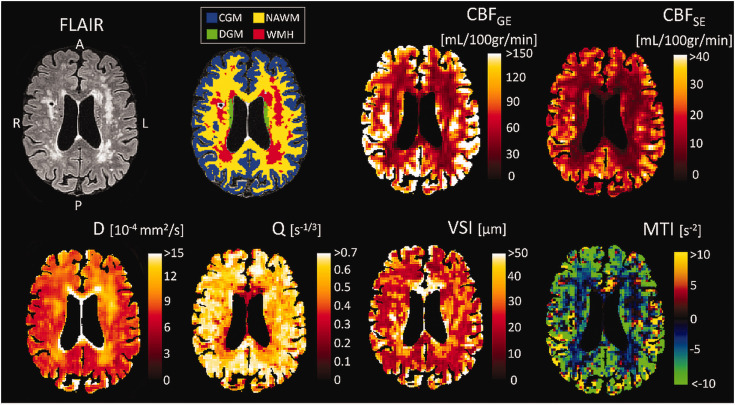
Examples of the MRI-derived images. Example of a FLAIR image, brain segmentation, cerebral blood flow derived from gradient echo (CBF_GE_), cerebral blood flow derived from spin echo (CBF_SE_), diffusion coefficient (D), vessel density index (Q), vessel size index (VSI), and microvessel type indicator (MTI) image for a cSVD patient. Both CBF maps depict higher perfusion in gray matter compared to white matter and hypoperfusion in white matter hyperintensities (WMH). D is highest in WMH, indicating microstructural damage. Q is lower and VSI is higher in WMH compared to surrounding white matter. Overall, the brain tissue has a negative MTI value, indicating a larger volume fraction of the venules/veins compared to arterioles/arteries. CGM: cortical gray matter; NAWM: normal-appearing white matter; DGM: deep gray matter.
